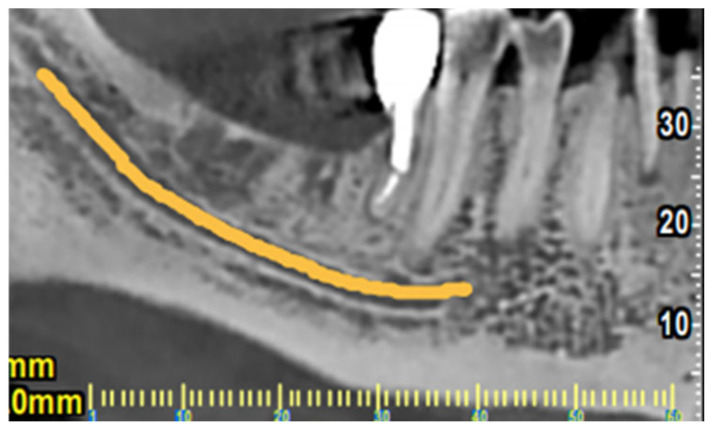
CBCT view of the lower right region of the mandible used to evaluate bone dimensions, bone quality, and the course of anatomical elements for implant placement (e.g., inferior alveolar nerve marked with orange). The retained mesial root of #46 remained functional after hemisection and crown restoration without signs of periapical pathology, root fracture or caries.
